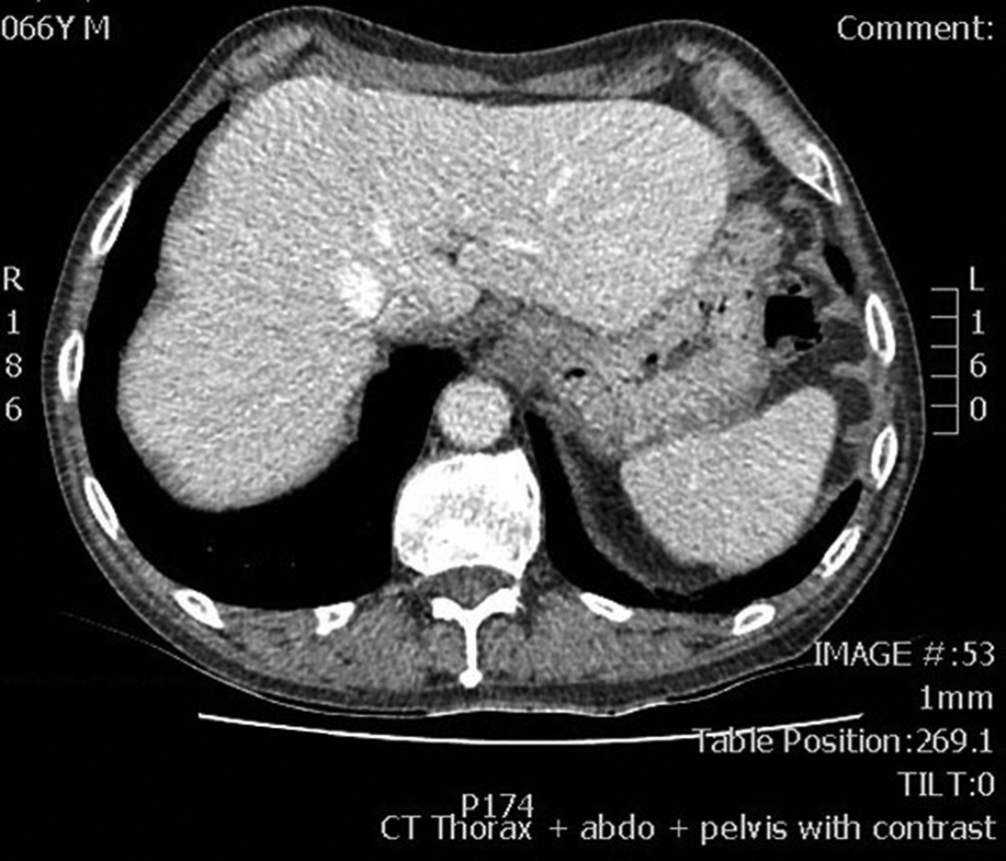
CT abdomen and pelvis January 2015 demonstrating a normal appearing stomach.
